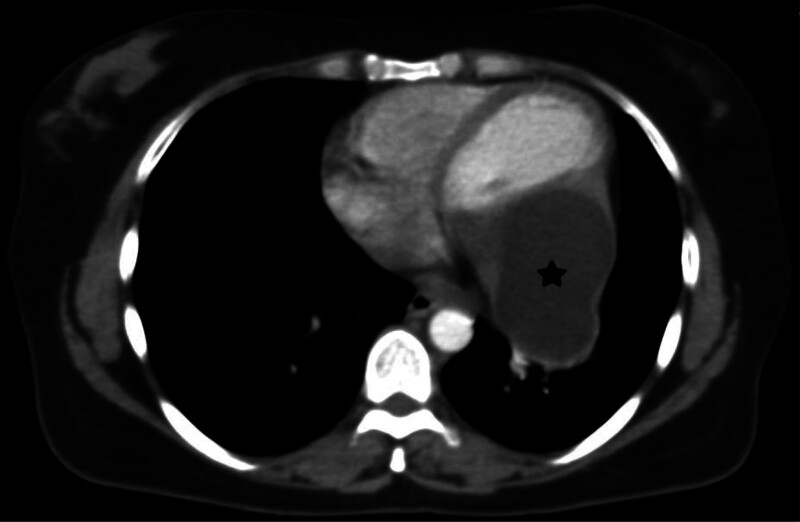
Axial contrast-enhanced CT scan shows the cardiac hydatid cyst at the left ventricular apex (black star). CT = computed tomography.
